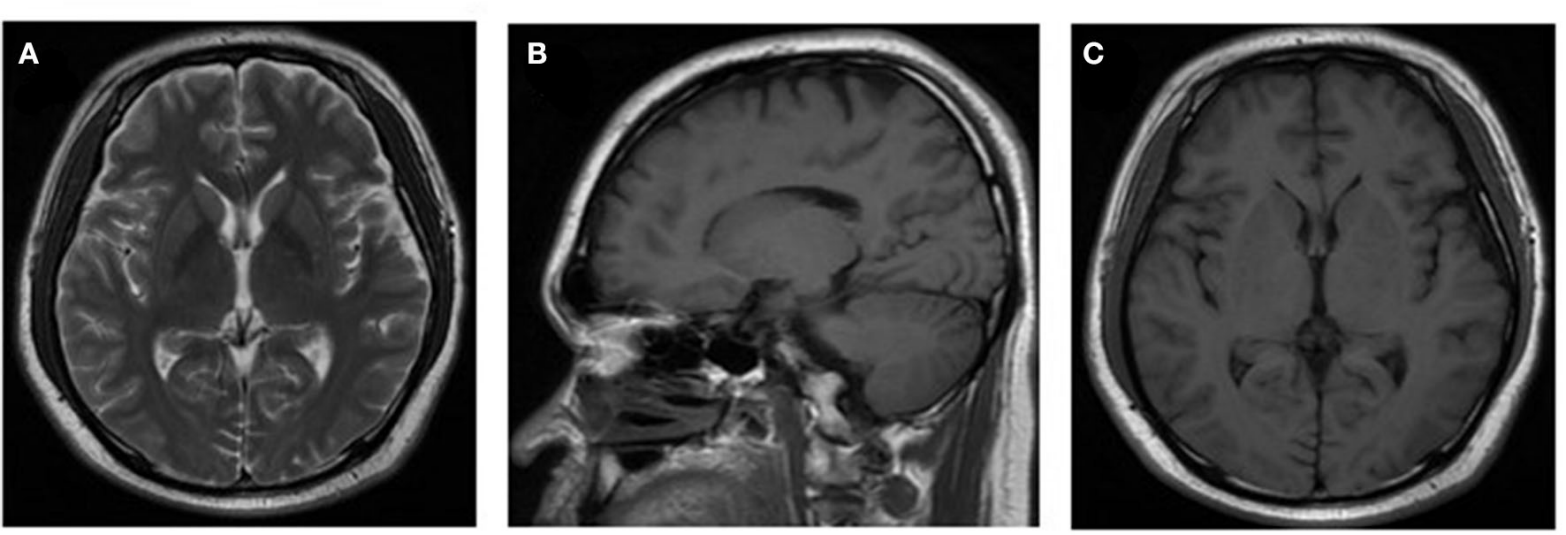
Neuroradiologic MRI. The MRI examination results of the patient were unremarkable (images shown in pictures A–C).
